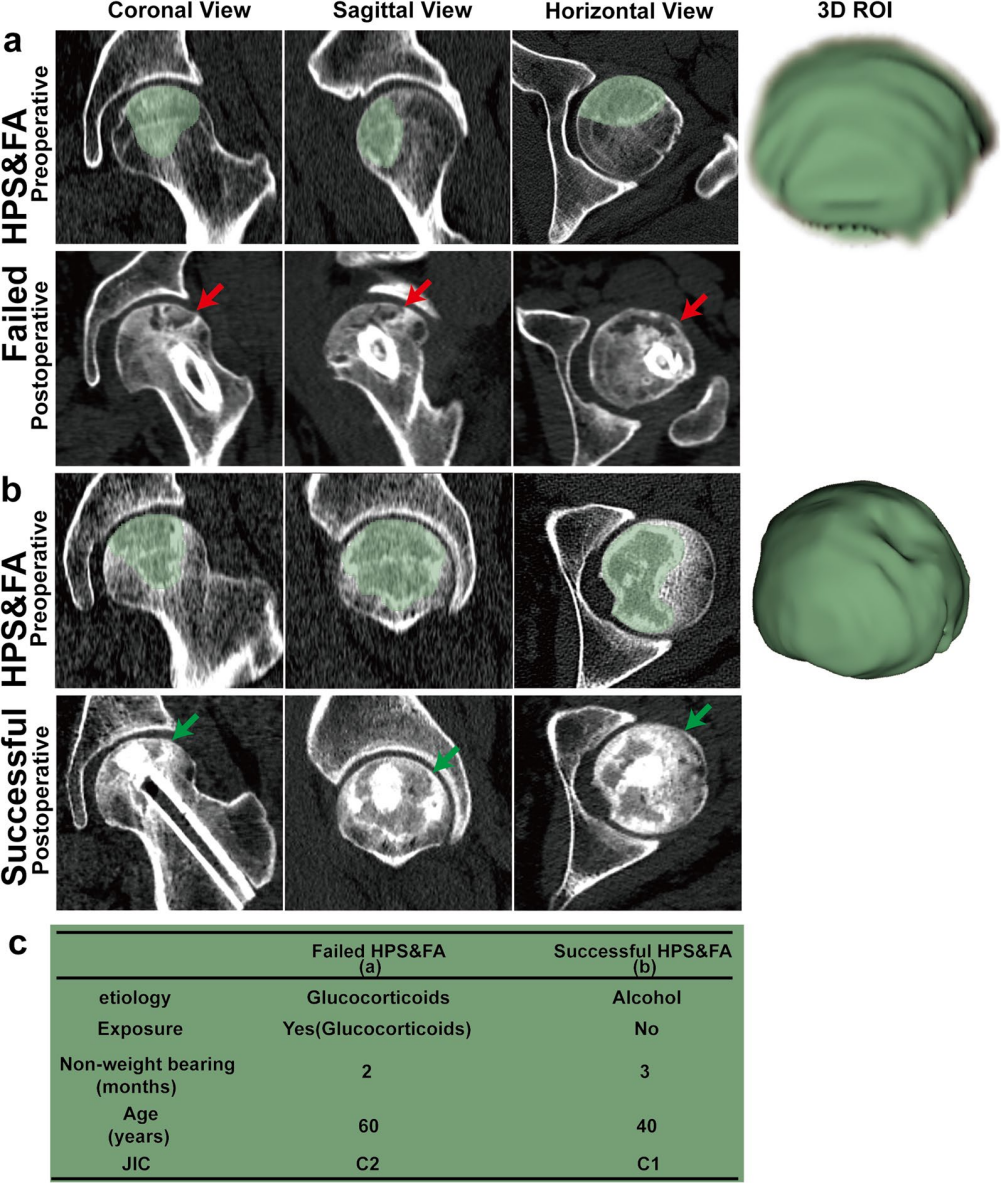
Representative CT images for failed a HPS&FA and successful b HPS&FA. c Comparison of basic information and risk factors. Red arrow: necrosis volume was large and involved the lateral column in (a). Green arrow: necrosis volume was relatively small and the lateral column was not accumulated in (b)
